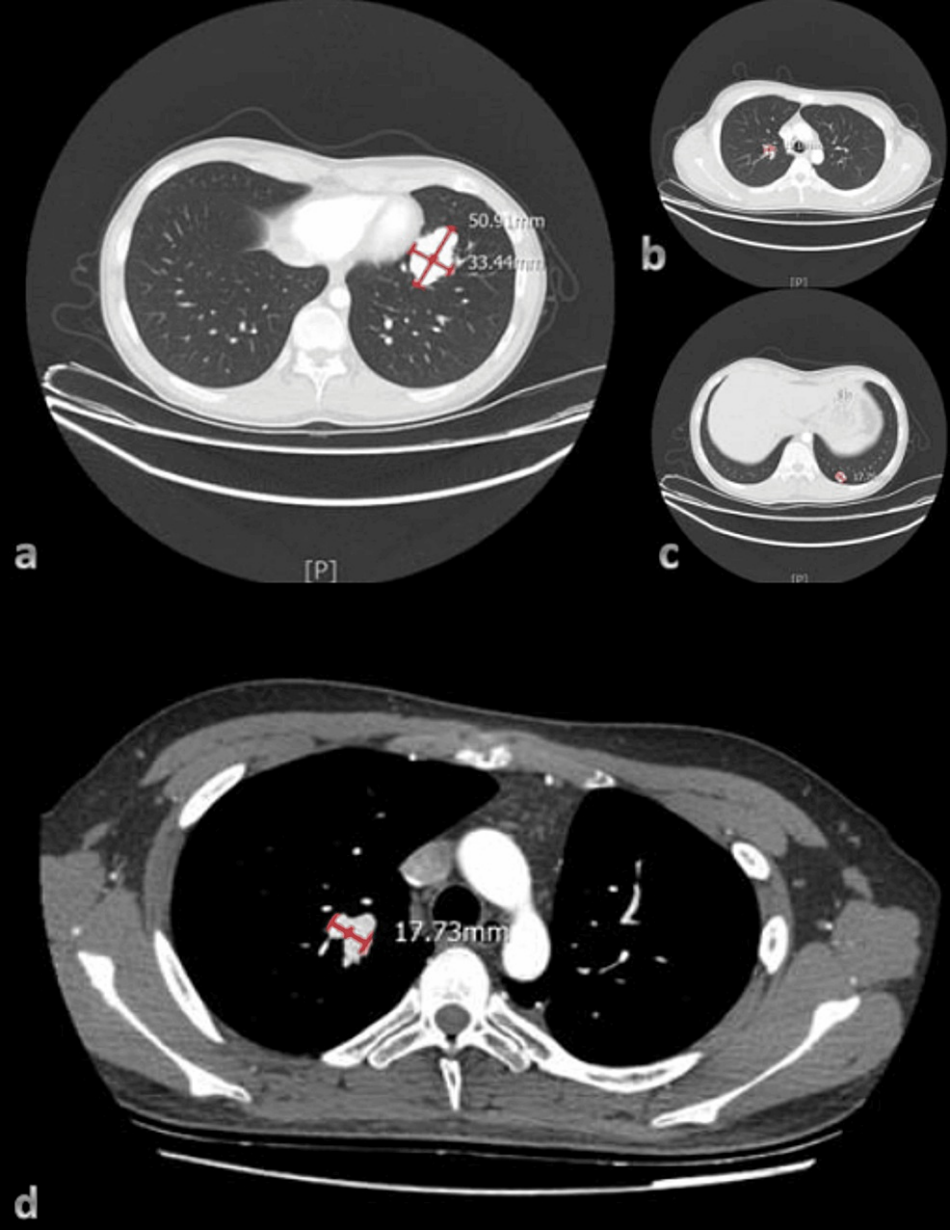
Arteriovenous fistulas were observed in the right upper lobe and left lower lobe of the pulmonary artery computer tomography angiography (CTA), with the following measurements: (a) 50.91 × 33.44 mm, (b) 17.75 × 9.66 mm, and (c) 19.10 × 8.36 mm. At six months after the operation, the arteriovenous fistula in the right upper lobe of the pulmonary artery CTA had a measurement of (d) 17.73 × 9.83 mm. (c) After resection of the left lower lobe and thoracic drainage, the left pneumothorax persisted at a similar level as before.
